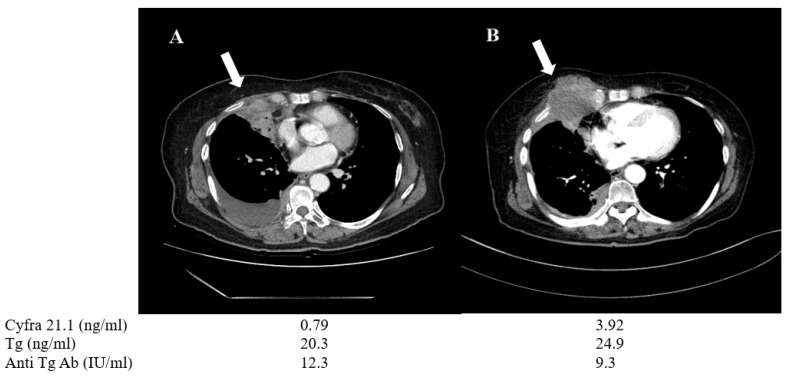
Changes in chest CT images and serum biomarkers of a 70-year-old PTC female (patient No. 6) before (A) and after one year of TKI treatment (B). During the time interval, the tumor progressed and changed to anaplastic feature (arrow).
